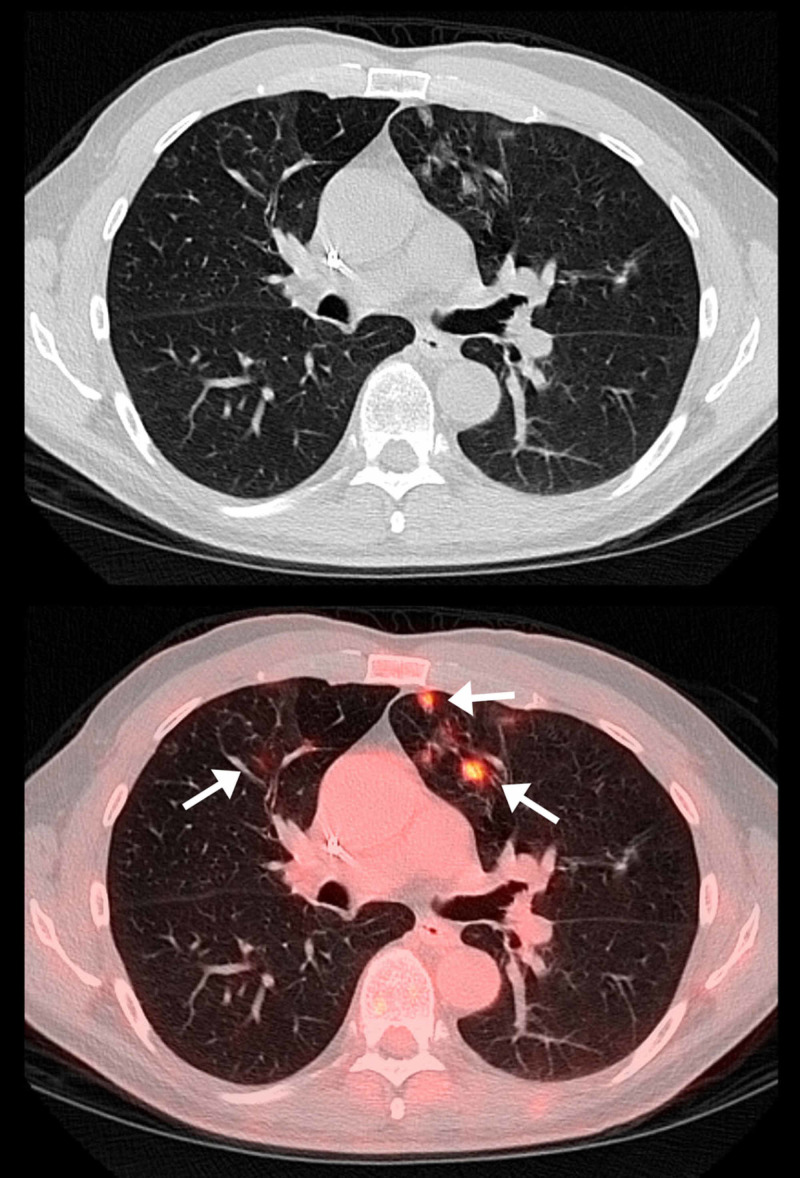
Selected axial CT and PET-CT fusion images. Selected axial CT and PET-CT fusion images demonstrate multiple, predominantly peripheral ground-glass opacities within the left upper lobe (arrow) with more subtle ground glass opacities in the right upper lobe with increased fluorodeoxyglucose (FDG) uptake (arrow).
